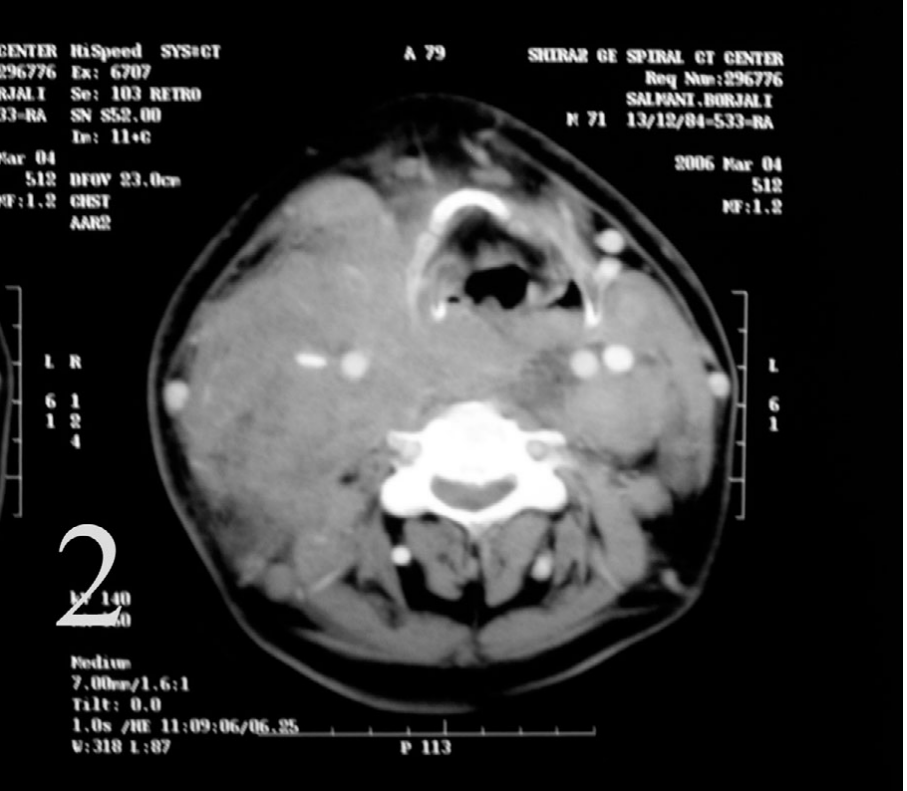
CT scan was performed which showed a large cervical mass arising form thyroid and extending to left cervical soft tissue with displacement of trachea.
